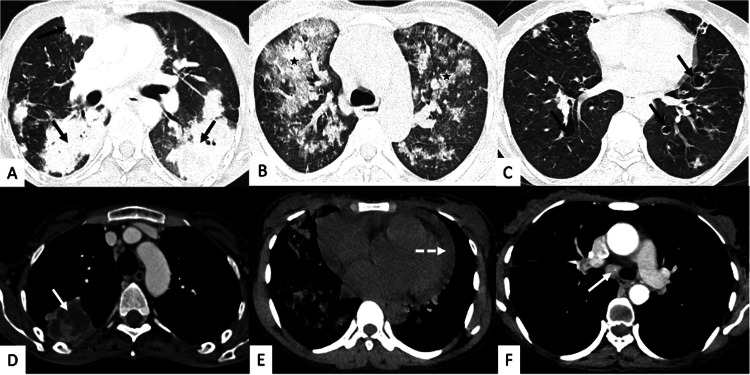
Computed tomography (CT) thorax in different patients Case 1: High-resolution CT (HRCT) (A) and contrast-enhanced CT (CECT) (D) showing a multifocal patchy area of consolidation (black arrows) with internal necrosis (white arrow). Case 2: HRCT (B) and noncontrast CT (NCCT) (E) showing diffuse consolidation and ground glass in bilateral lungs (asterisk) with pericardial effusion (dashed arrow). Case 3: HRCT (C ) and CECT (F) showing bronchiectasis (black arrows) with multiple parenchymal nodules and mediastinal lymphadenopathy (white arrow).
